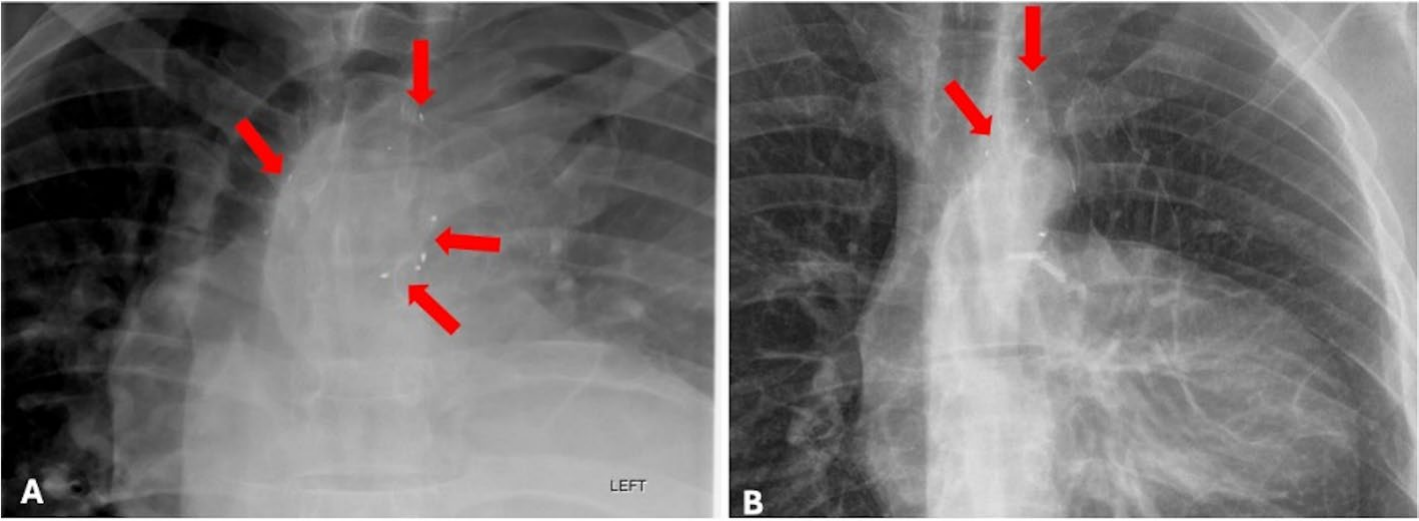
A Immediate post-embolization on-table radiograph demonstrating plug occluder positions (red arrows). B The 2-month follow-up chest radiograph demonstrating unchanged positioning of the unresected plug occluders
